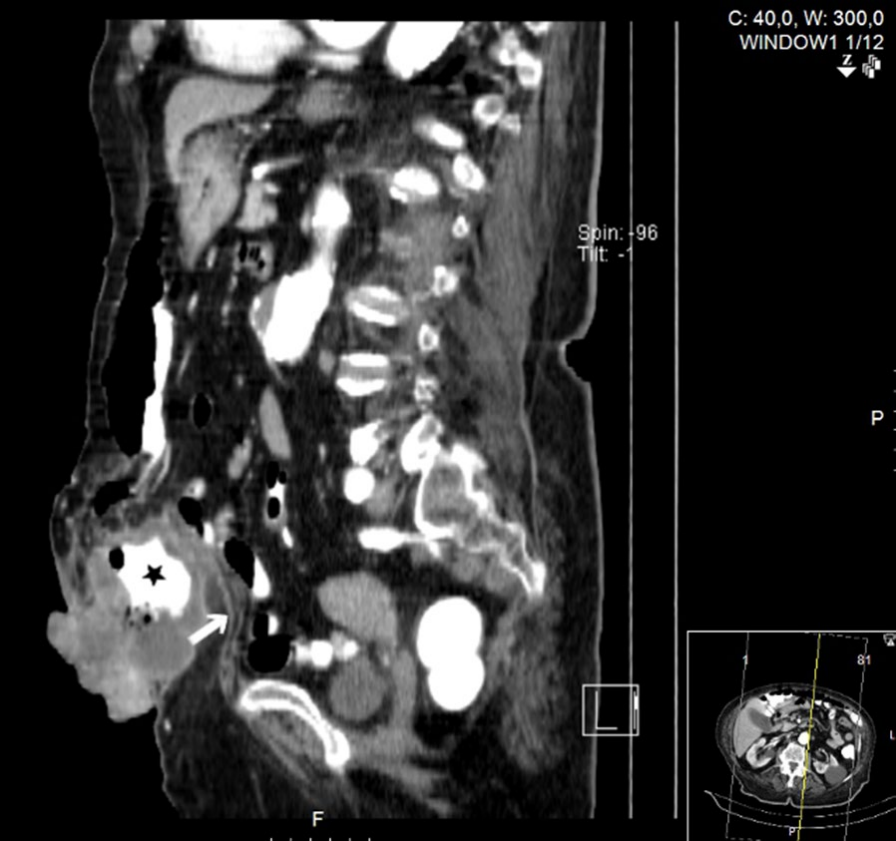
Computed tomography: note the presence of the transverse colon (*star*) within the hernia sac, outside the muscular layer of the abdominal wall (*white arrow*)
